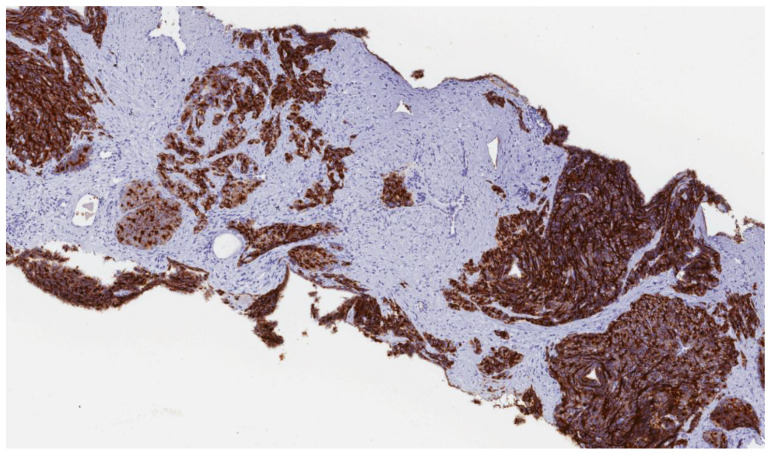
Biopsy sample of prostate tissue infiltrated by prostatic adenocarcinoma, with a strong and diffuse prostate specific membrane antigen (PSMA) expression. (magnification: 4×)
